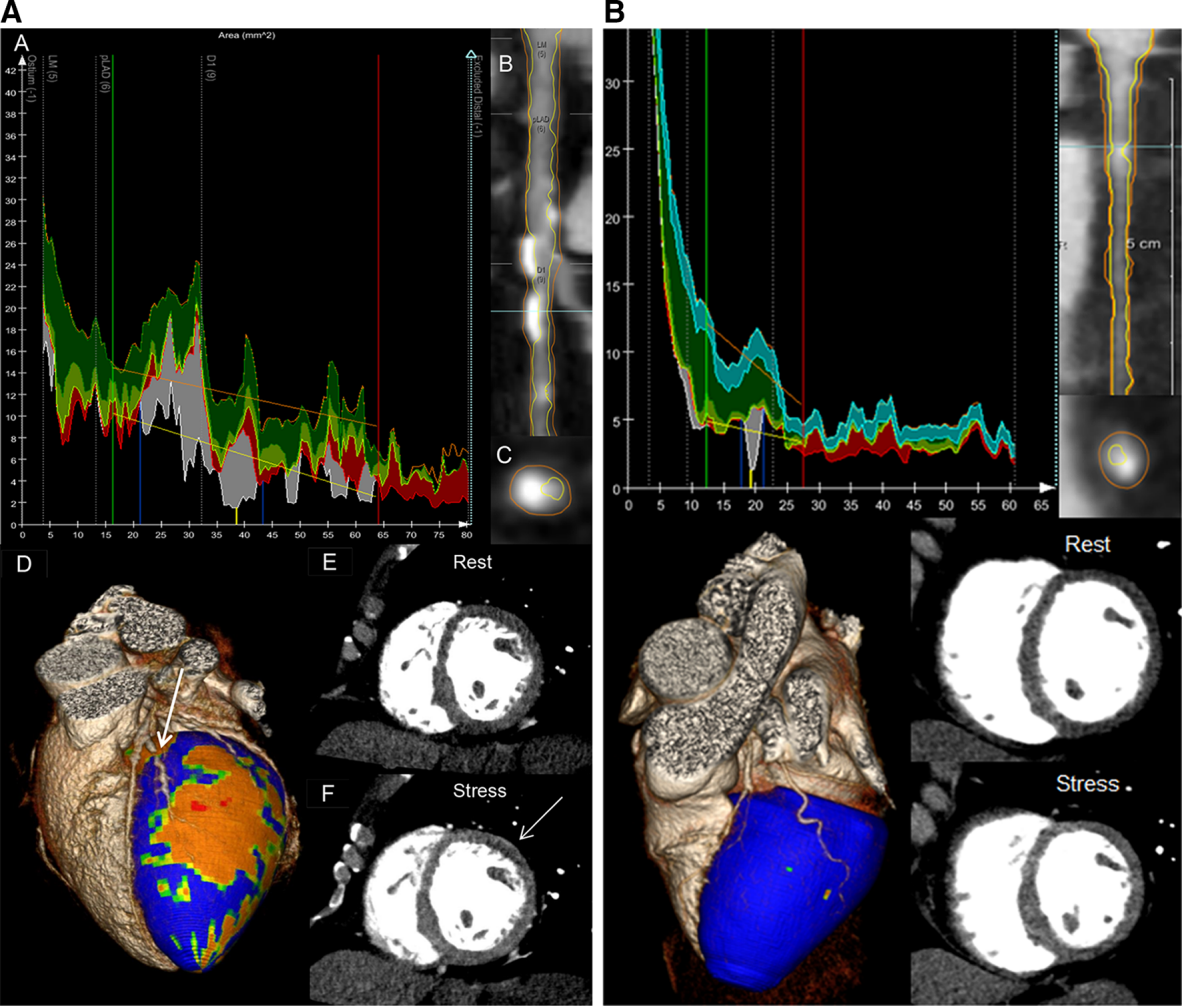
(A) Quantitative CTA and adenosine stress CTP of an ischemic lesion. Example of a 58-year-old female patient with a lesion in the first diagonal branch and corresponding myocardial ischemia. *A* Automated quantitative CTA of the artery was performed. The *blue lines* were set proximal and distal to the lesion. The *green* and *red lines* represent non-diseased coronary artery segments proximal and distal to the lesion. The *yellow* and *orange lines* represent the reference markers for, respectively, the lumen and vessel wall. The *vertical yellow line* is placed at the site of maximal stenosis percentage: 76.6%. Furthermore, mean plaque burden was 79.9%; plaque volume: 129.3 mm^3^; lesion length: 19.0 mm; maximal plaque thickness: 2.6 mm; dense calcium volume: 36.6 mm^3^. *B* Longitudinal lumen and vessel wall contours. *C* Transverse lumen and vessel wall contours at the site of maximal stenosis percentage. *D* 3D fusion of the coronary CTA and myocardial hypo-perfusion during adenosine stress (*orange*, *red*). A stenosis in the first diagonal branch (*arrow*) is depicted with corresponding myocardial ischemia. *E* Rest CTP study showing normal myocardial enhancement. *F* Adenosine stress myocardial CTP showing a small anterolateral subendocardial perfusion defect (*arrow*). (B) Quantitative CTA and adenosine stress CTP of a non-ischemic lesion. Same data reconstructions as shown in (A). A non-ischemic coronary lesion in the proximal LCX is depicted. Maximal stenosis percentage was: 69.1%. Mean plaque burden was: 67.2%; plaque volume: 27.1 mm^3^; lesion length: 3.5 mm; maximal plaque thickness: 1.8 mm; dense calcium volume: 9.4 mm^3^. Despite the high stenosis percentage, lesion length, maximal plaque thickness, and dense calcium volume were relatively low, resulting in normal myocardial enhancement on adenosine stress
